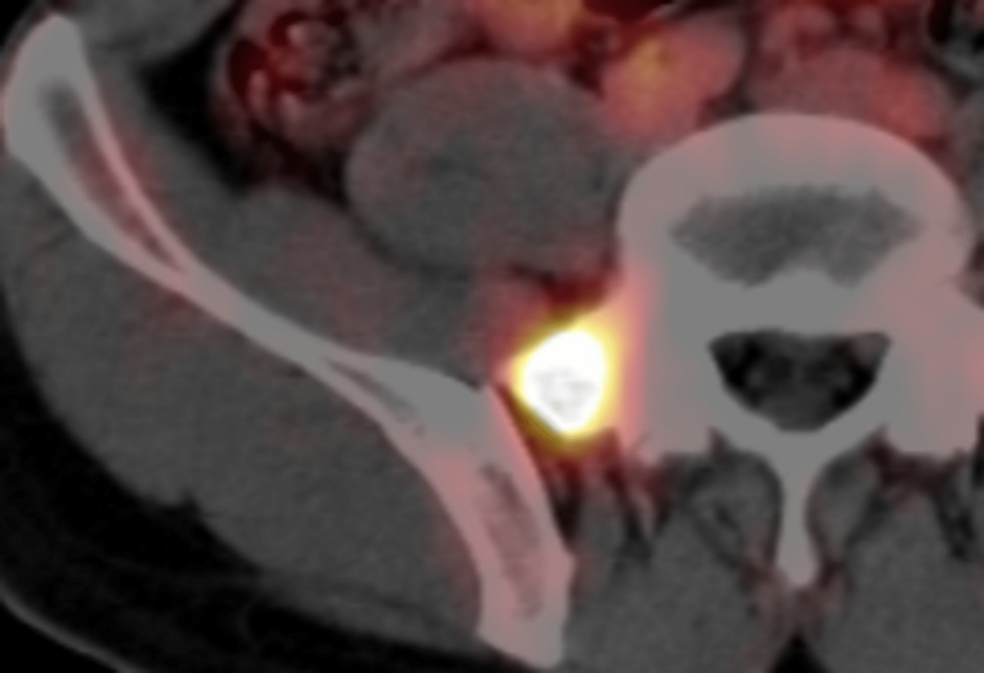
18F-FDG PET/CT imaging before the chemotherapy
